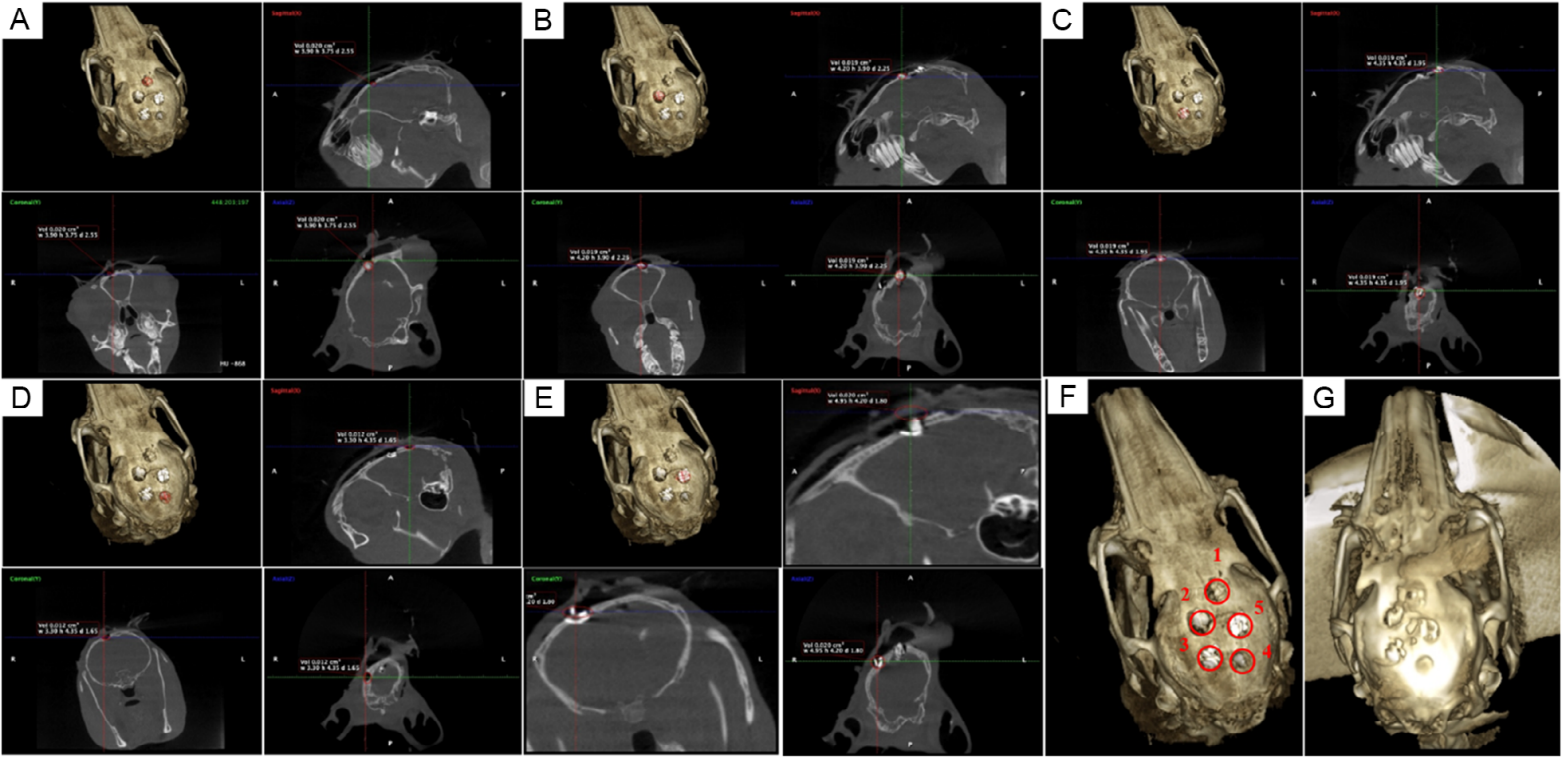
CBCT images of the cranial defect cavity at 7 and 28 d, shown in coronal, axial, and sagittal views using Romexis Viewer software: (A,B) Undoped 58S bioglass at 28 days, (C,D) Sr/Mg‐doped 58S at 28 d, (E) Untreated cavity as the control sample at 28 d, (F) Numbering of cavities and cranial defect cavity at 7 d, and (G) cranial defect cavity after treatment at 28 d post‐implantation.
